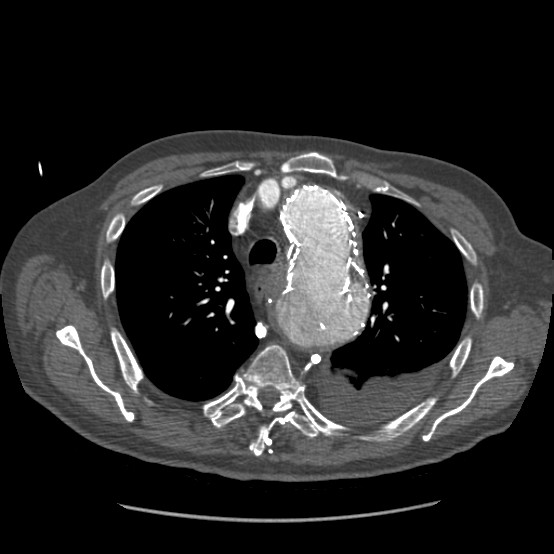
Two dimensional CT image showing posterior defect in the thoracic stent component and associated haematoma in the left thoracic cavity.
